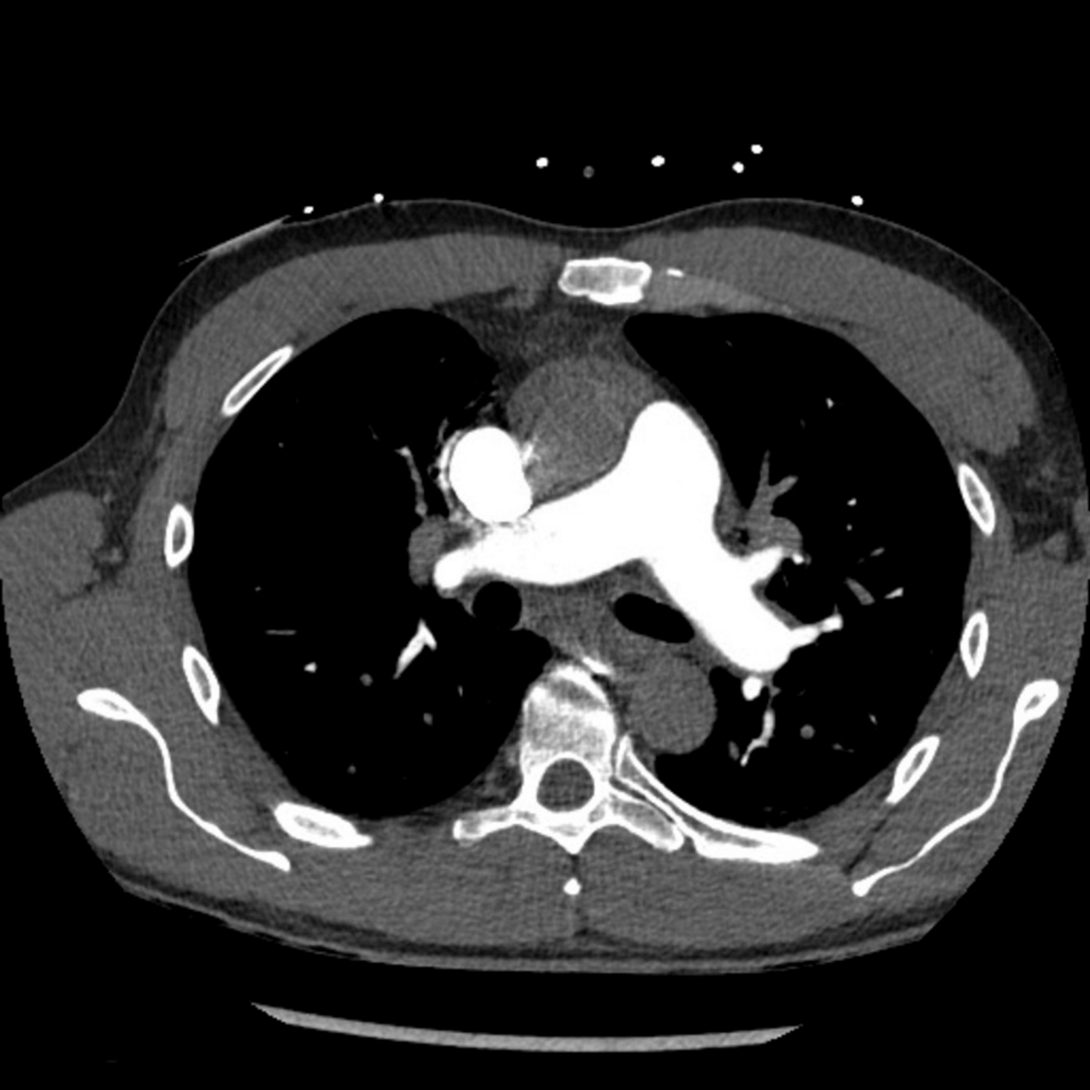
Computed tomographic pulmonary angiography (CTPA) showed no pulmonary embolism.
